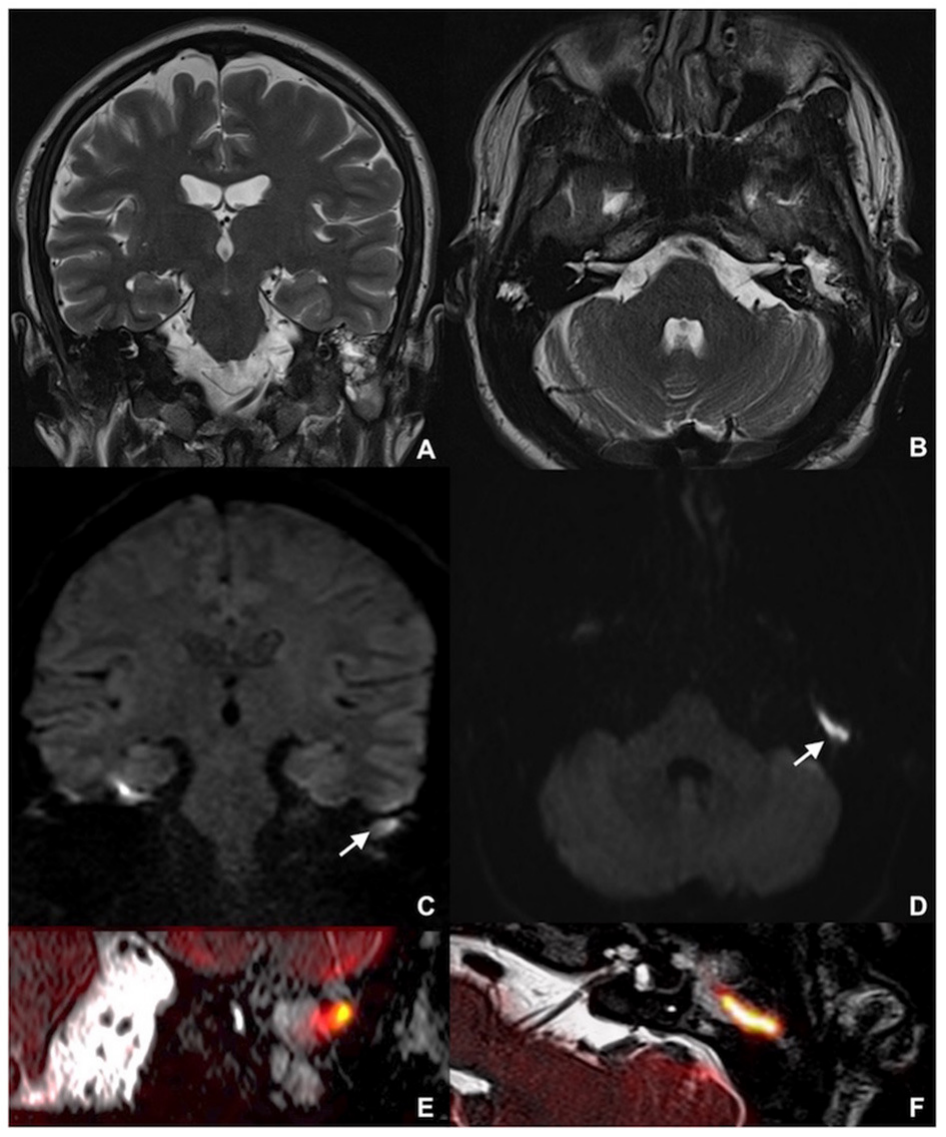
MRI showing left middle ear recurrent cholesteatoma in a 26-year-old man: coronal (A) and axial (B) TSE T2w; coronal MSH non-EPI DWI (C) and axial SE-EPI DWI (D); detailed coronal (E) and axial (F) fusion imaging of both ultra-thin heavily-weighted 3D T2w sequences and DWI images. MRI, magnetic resonance imaging; TSE, turbo spin echo; MSH, multi-shot; EPI, echo planar imaging; DWI, diffusion weighted imaging; SE, spin-echo.
